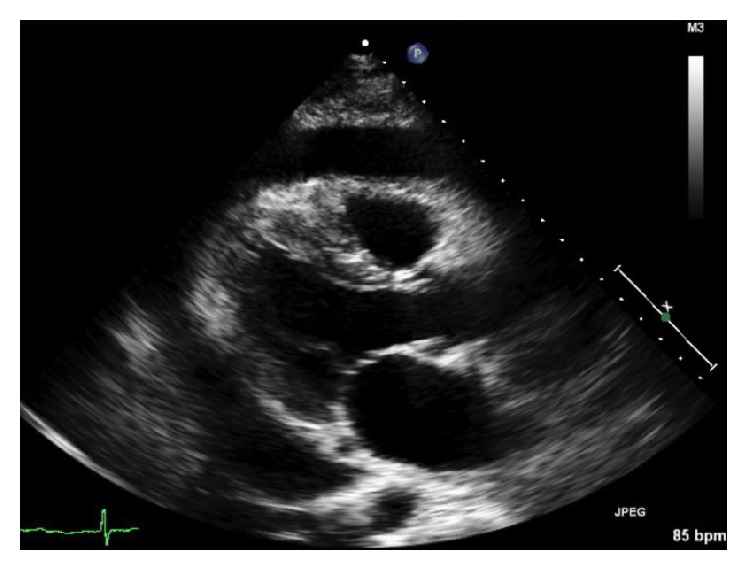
Transthoracic echocardiography (TTE) performed on day one of the hospitalization. The image shows a pericardial effusion.
